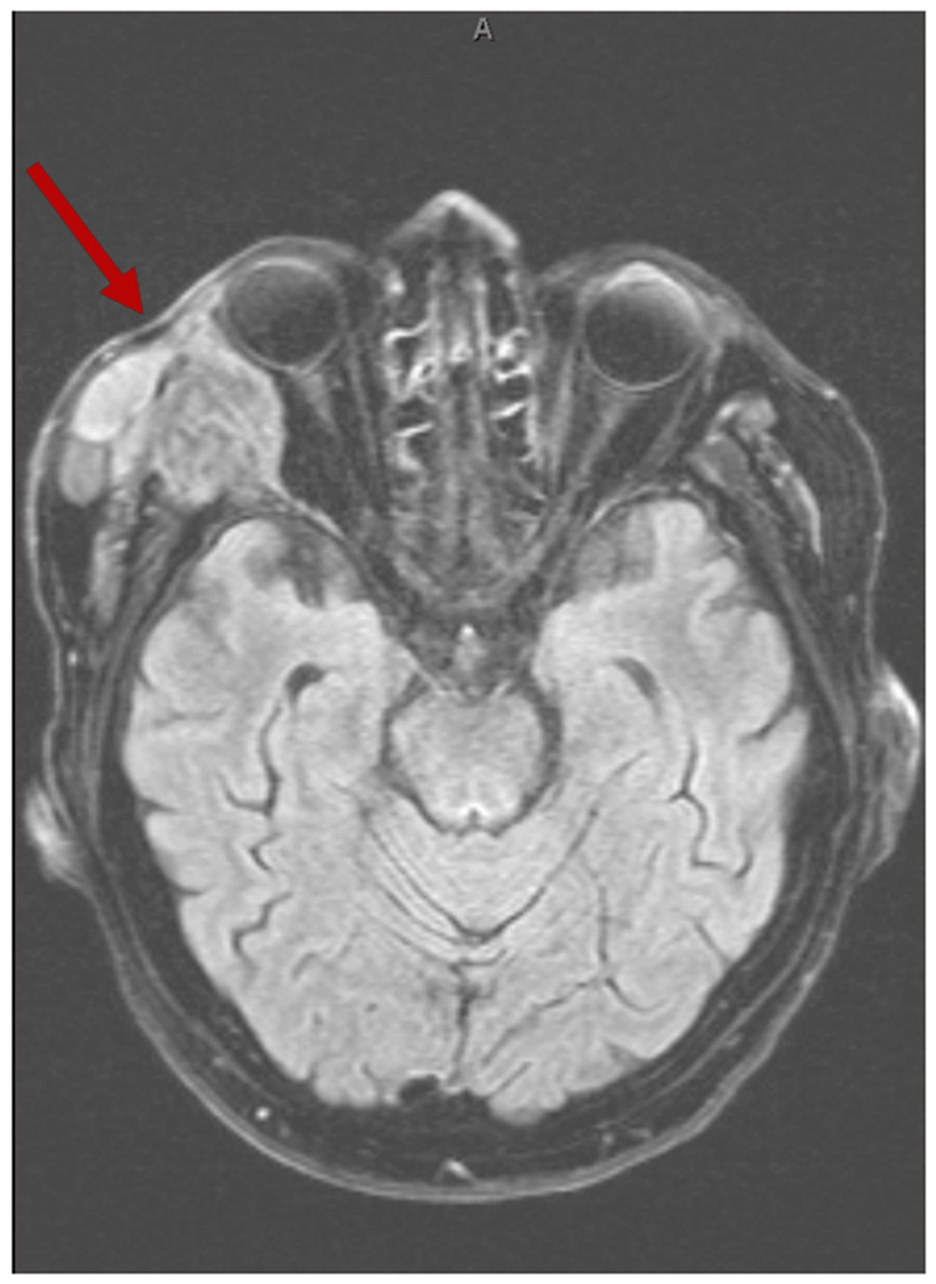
Magnetic resonance imaging (MRI) of the brain, face, orbits, and neck showing lesions in the right orbital region (shown in the arrow).
